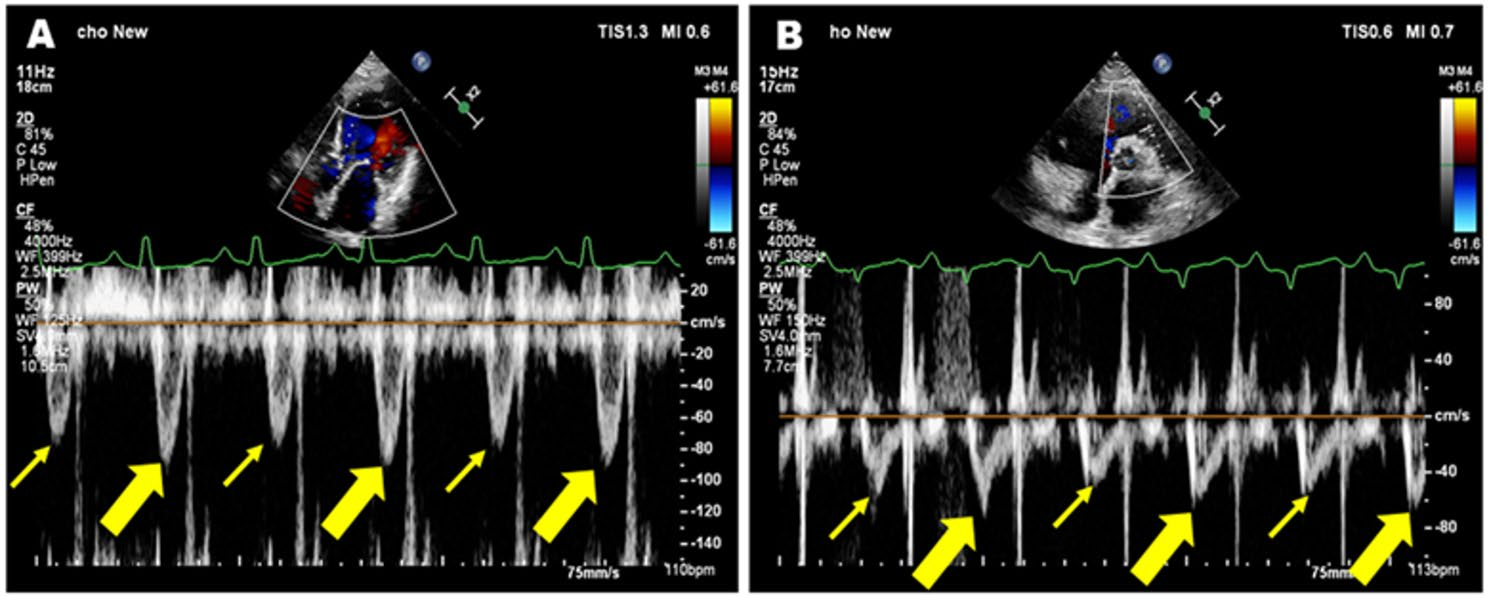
Pulsed-wave Doppler demonstrating a patient with biventricular PA. A The strong beat (bold yellow arrow) and the weak beat (thin yellow arrow) in the left ventricular outflow tract; B The strong beat (bold yellow arrow) and the weak beat (thin yellow arrow) in the right ventricular outflow tract
